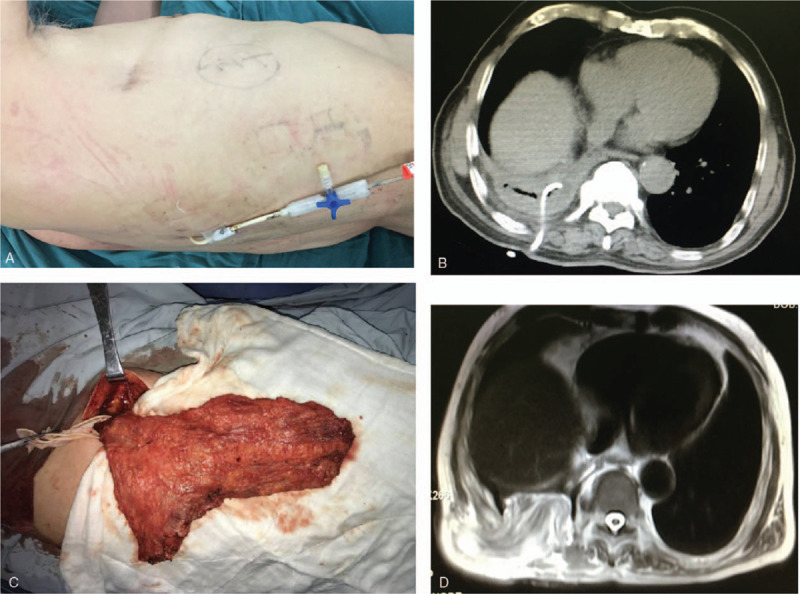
Clinical data of patient 1. A, A preoperative image revealed a uniportal incision and a drainage tube in the right lower thorax. B, A preoperative chest computed tomography (CT) scan showed a empyema cavity containing pleural effusion in the right lower thorax. C, During the operation, a 15 × 11 cm pedicled latissimus dorsi muscle flap was harvested and the tip of the pedicled muscle flap was sutured and fixed to the anterior lower mediastinum and the rest was transposed to completely obliterate the empyema cavity. D, A postoperative chest magnetic resonance imaging (MRI) scan showed successful obliteration of the empyema cavity and bronchopleural fistula BPF after surgery.
